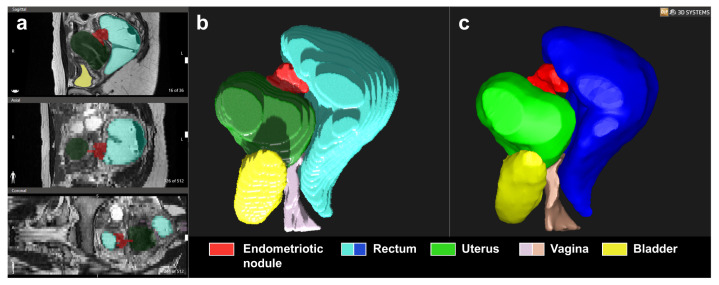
Display of the process of obtaining the 3D virtual anatomical model (c) by segmentation of the anatomical regions of interest (b) based on the magnetic resonance imaging (MRI) scan (a), using D2P^TM^ software (3D Systems).
